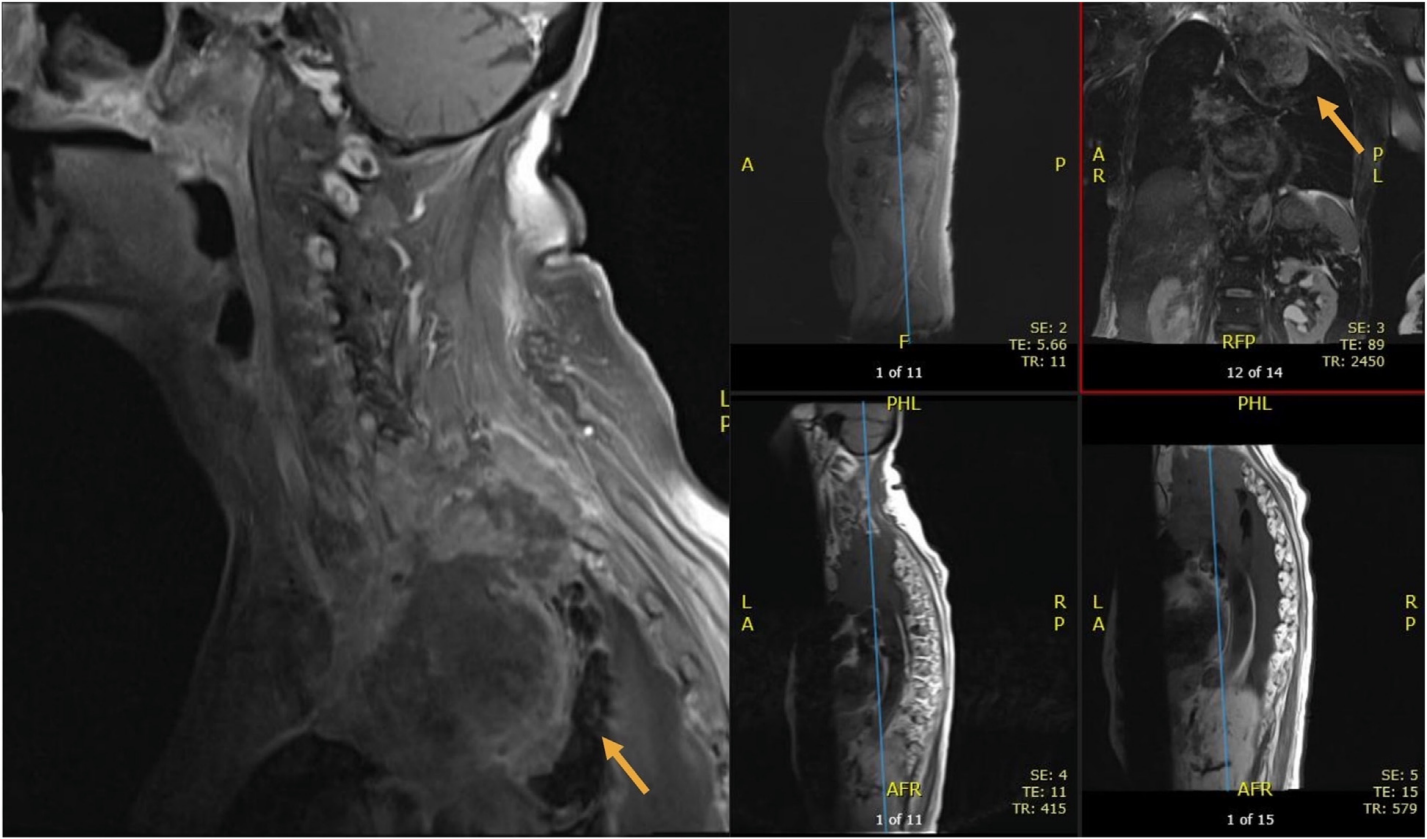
Thoracic MRI demonstrating a left upper lung lobe mass measuring 11.0 cm with encasement of the left common carotid artery and posterior elements of T1 and T2 segments. *MRI*, Magnetic resonance imaging.
